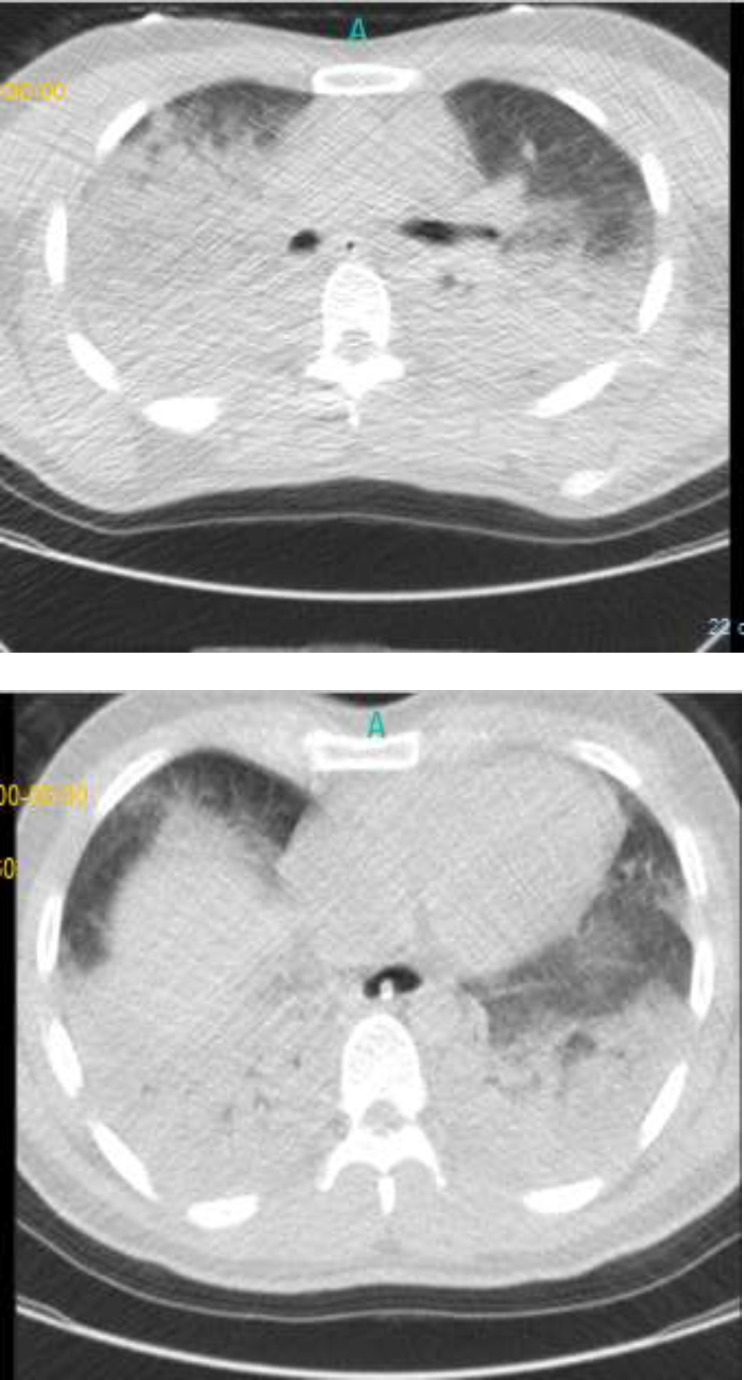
Chest CT-scans of case-patient with COVID-19 on March 27, 2020 at the onset of the disease
